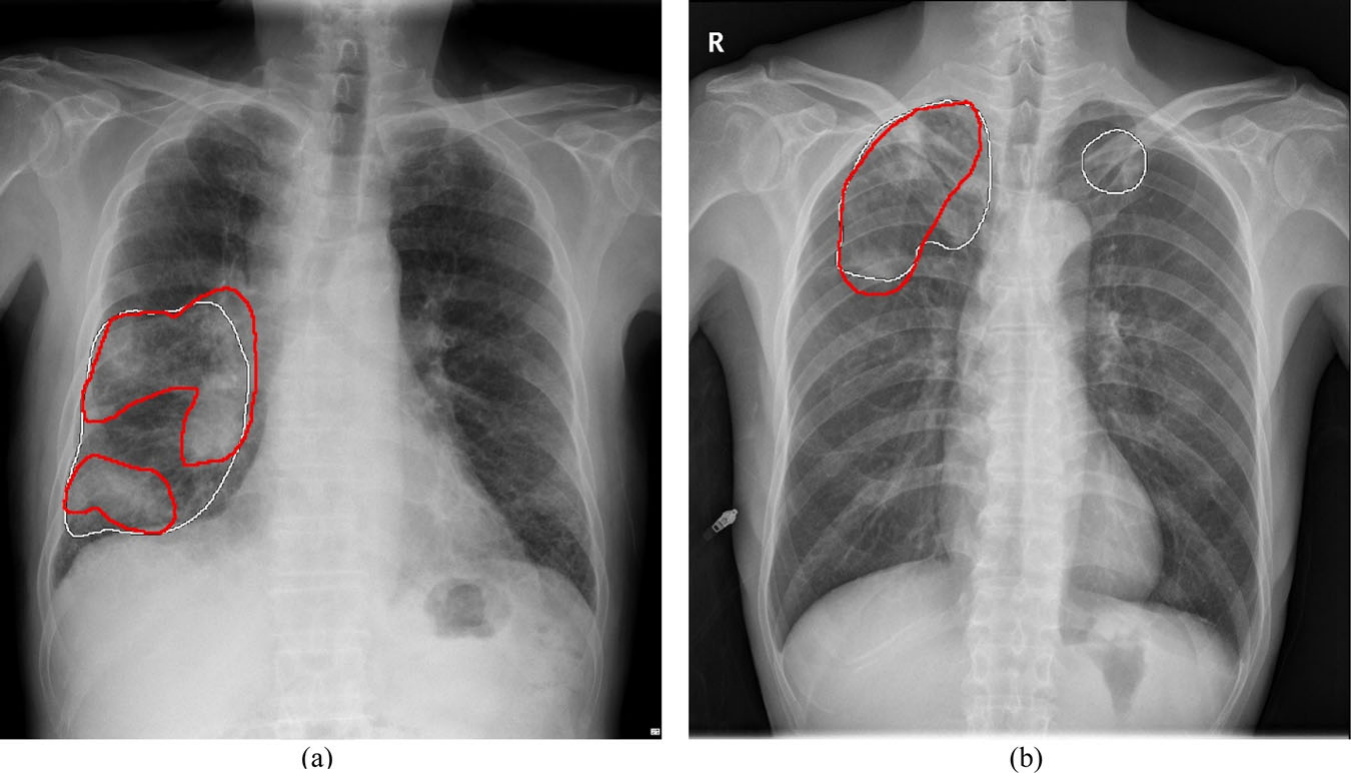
Examples of CAD predictions on chest radiographs. a Closely located and diffuse lesions are predicted as a single large lesion, and b dense bone structures such as the intersection of the rib and clavicle or sternum were falsely identified as consolidation. CAD Computer-aided detection system.
